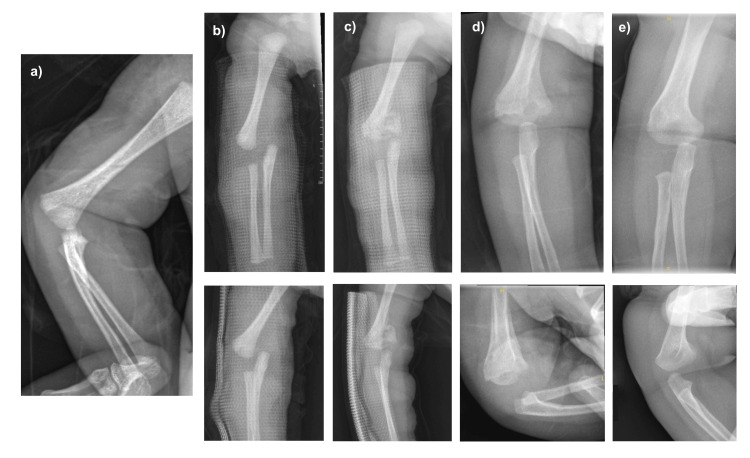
Serial elbow radiographs of case 3 Serial elbow radiographs at: (a) initial injury: showing medial displacement of the forearm axis compared to distal humerus and the oblique view presents a diagnostic difficulty; (b) day 5: showing medial displacement of the forearm axis in relation to the distal humerus; (c) 3 weeks: showing callus formation; (d) 5 weeks: showing a united fracture; (e) 4 months: showing remodelling of the distal humerus.
